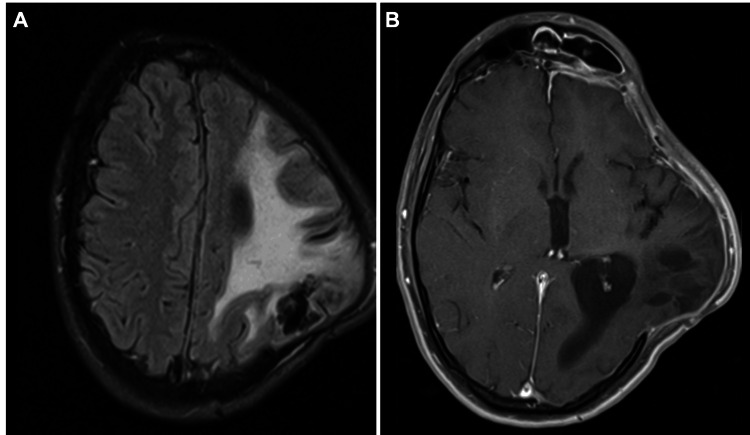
Contrast-enhanced cranial MRI six months post-admission. (A) Axial FLAIR with substantial cortico-subcortical encephalomalacia causing a slight ipsilateral midline shift. (B) Axial T1 fat-saturated contrast-enhanced image with no signs of subdural empyema relapse. Passive left ventricle enlargement (occipital horn) and chronic left frontal sinusitis can be noted. FLAIR: fluid-attenuated inversion recovery; MRI: magnetic resonance imaging
